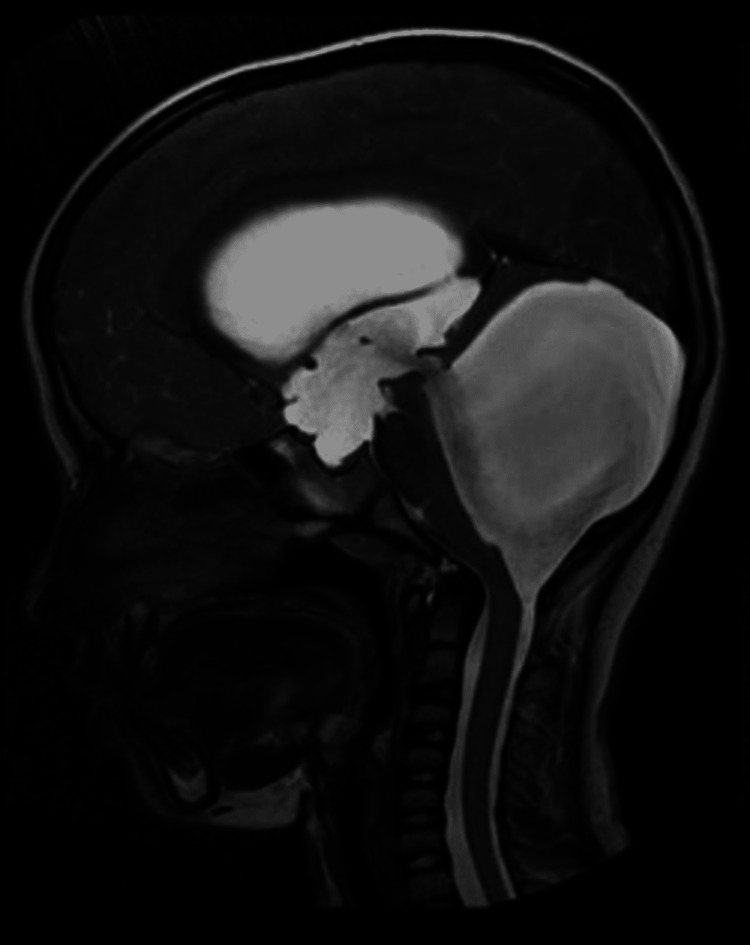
Brain MRI plain sagittal T2-weighted image shows dilated ventricular system with the fourth ventricle communicating with a large posterior fossa cyst compressing the brain stem anteriorly and pushing the hypoplastic cerebellar hemisphere superiorly.
